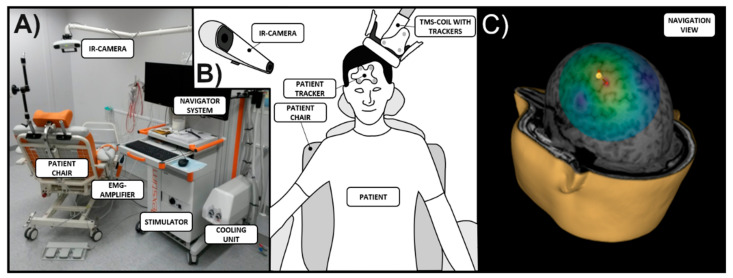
Setup for nTMS mapping. (A) Hardware of the nTMS system, including an infrared camera, EMG amplifier, neuronavigation monitor, and the stimulator with a cooling unit as the central components. (B) Patient positioning during mapping procedures, requiring initial co-registration of the patient’s head with cranial MRI (using a head tracker attached to the patient’s forehead) to be able to track the stimulating coil (equipped with infrared trackers) in relation to individual brain anatomy. (C) Navigation view during the mapping procedure, showing the stimulating coil (*yellow marker*) with its orientation (*red arrow*) and modelled EF distribution.
